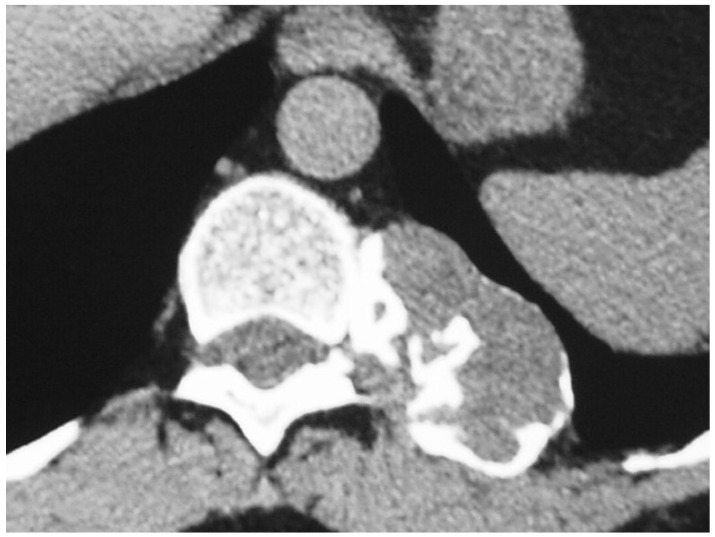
A 62-year-old female patient with grade 2 chondrosarcoma at the posterior rib adjacent to the costovertebral junction. Axial chest CT shows a soft tissue mass at the posterior rib with calcifications.
